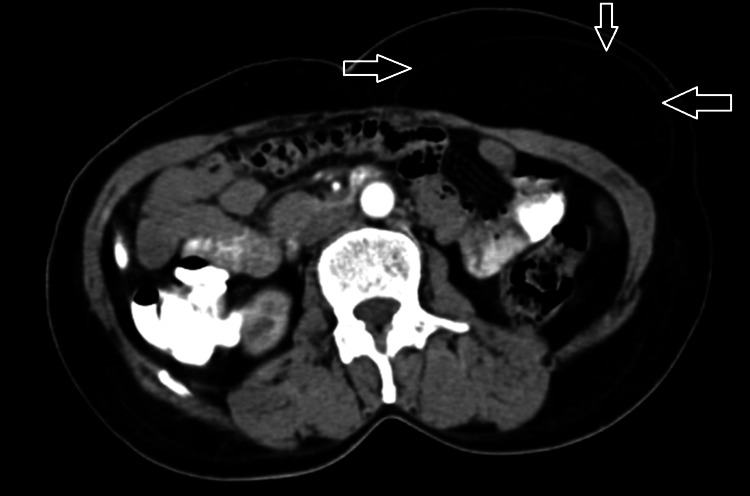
A computed tomography (CT) scan shows an encapsulated mass consistent with a lipoma, as indicated by arrows.
